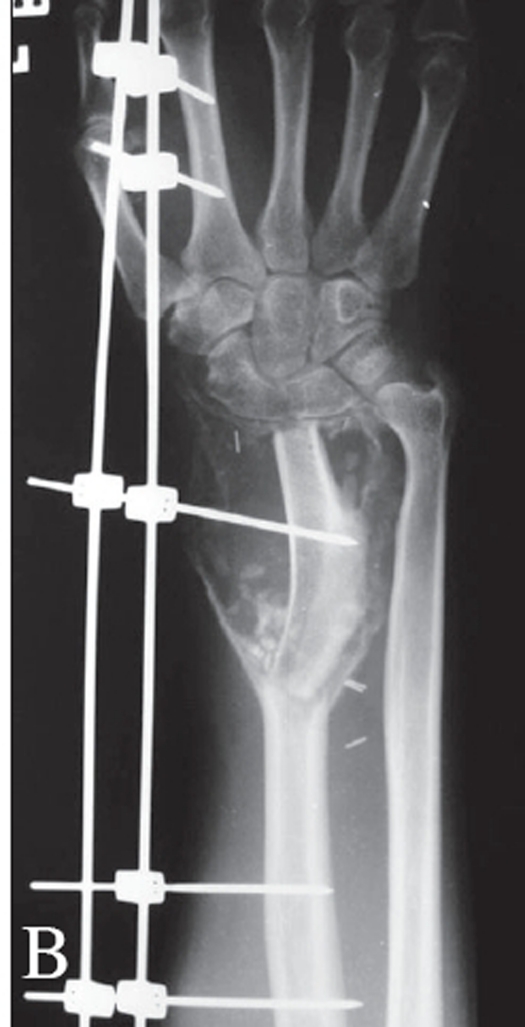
X-ray of the same patient treated with extended curettage and reconstruction with iliac crest strut bone graft along with morsellised grafts. Stabilization was achieved by an external fixator
